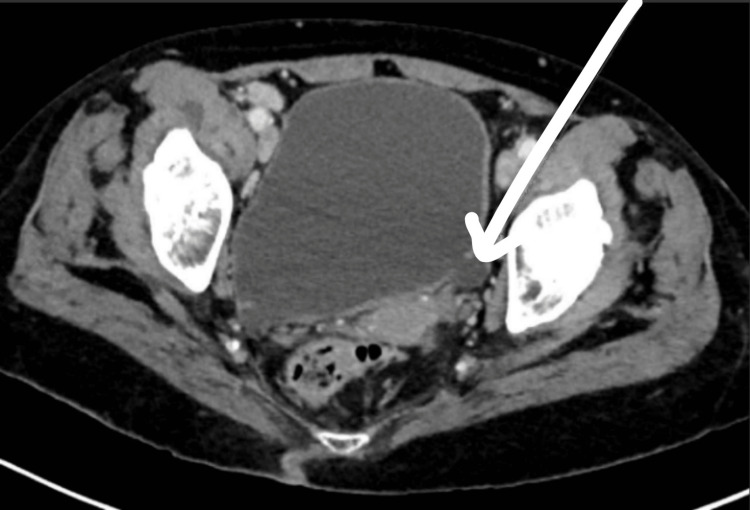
CT abdomen and pelvis with IV contrast showing a left small bladder diverticular; white arrow point inserted to show the mucosa outpouching of the bladder wall. Arrow pointing to the area of the diverticula
